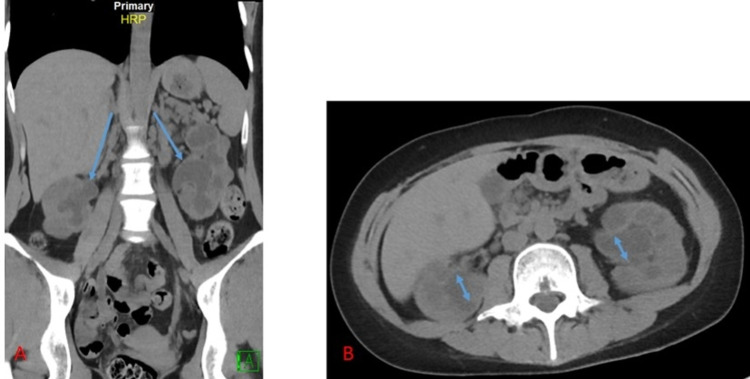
Non-contrast CT abdomen pelvis demonstrating ureteric wall thickening with hydroureteronephrosis (blue arrows), without nephrolithiasis.
